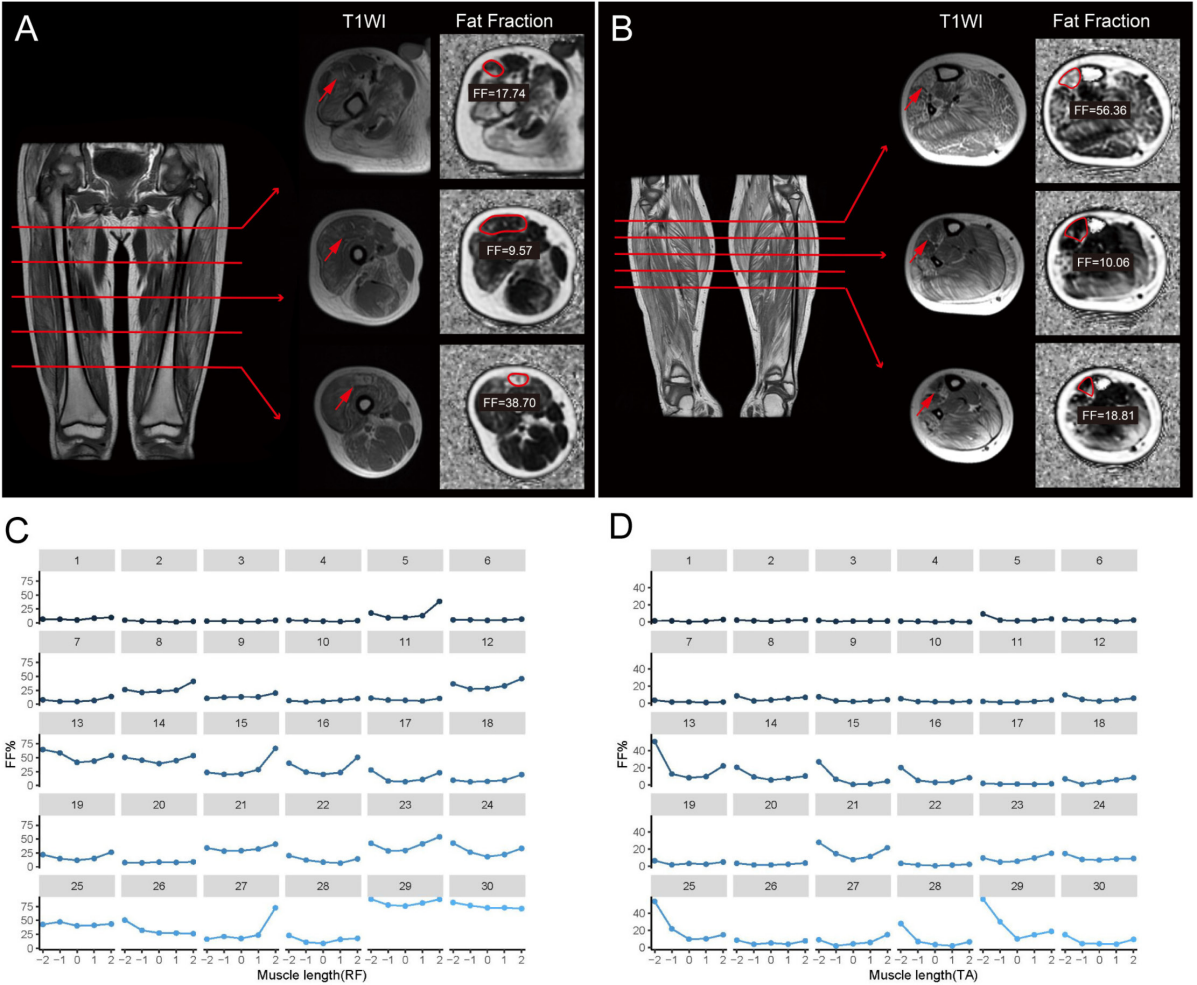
Fat distribution profiles along the full length of the muscles in DMD patients. For the (A) RF and (B) TA, three axial T1WI and IDEAL MRI slices were presented, elucidating the non‐uniform distribution of fat replacement along the proximodistal muscle axis of RF and TA. These two muscles were highlighted with manually drawn ROIs in IDEAL‐IQ and indicated with red arrows in T1WI. The coronal images provided an overview of the locations of the axial slices. (C,D) The diverse fat replacement patterns observed in RF and TA. The maxCSA was centred at ‘0’ on the *x*‐axis, with the proximal and mild‐proximal portions shown to the left (‘−2’, ‘−1’), and the distal and mild‐distal parts shown to the right (‘2’, ‘1’). Breakdown of the first 30 individual DMD patients was presented in each thumbnail plot. Filled circles represent FF values at different slices, connected by solid lines. A consistent muscle‐specific pattern of fat replacement was evident, observed in the majority. FF, fat fraction; maxCSA, maximum cross‐sectional area; RF, rectus femoris; ROI, region of interest; T1WI, T1‐weighted image; TA, tibialis anterior.
